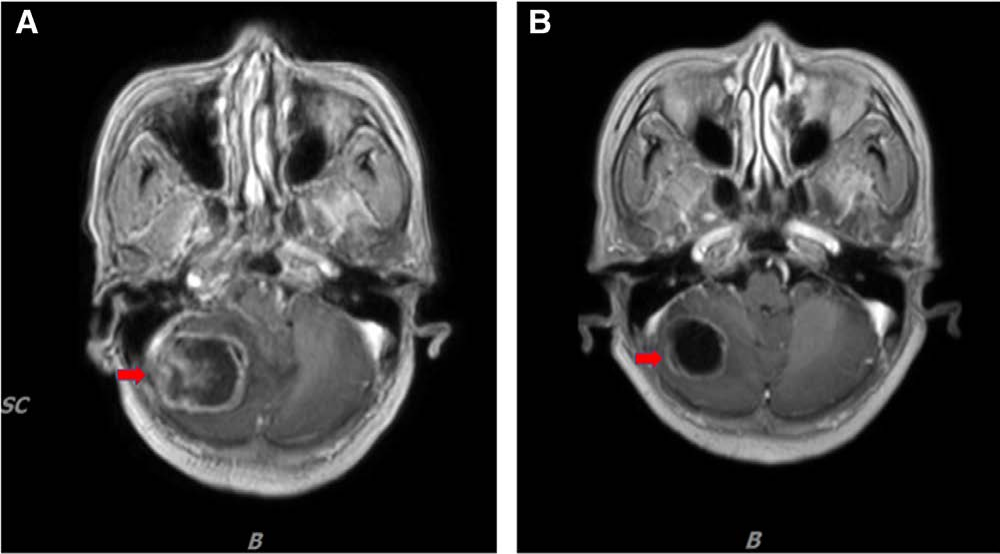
MRI images. Pre- and post-radiotherapy MRI scans reveal brain metastasis, with the lesion indicated by a red arrow. MRI = magnetic resonance imaging.
